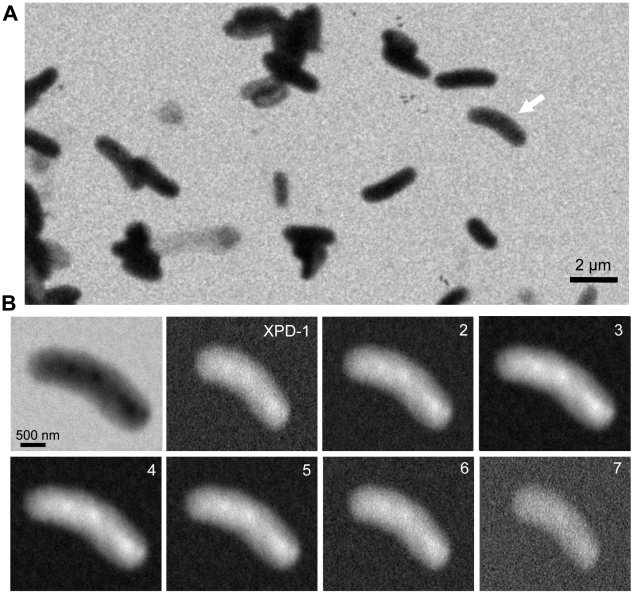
X-ray image of atmospheric unstained bacteria by SGXM system. (A) X-ray image of the atmospheric *Rhodobacter capsulatus* acquired by the photoconversion system using an original SE detector. The image was acquired at 4000× magnification at a 5-kV EB acceleration voltage. The image was filtered by a 2D Gaussian filter (size 15×15 pixels; σ = 1). (B) High-resolution X-ray images of the bacterium indicated by white arrow in panel (A). The top left image shows an X-ray image of a bacterium acquired with the SE detector and taken at 30,000× magnification. The X-ray images from XPD 1–7 were acquired by the linear PD array. Each X-ray image was corrected for the view angle and X-ray absorbance. In these images, XPD-1 is the tilt image at −40.2°, XPD-4 is placed approximately under the sample (4.4°) and XPD-7 is at 45°.
